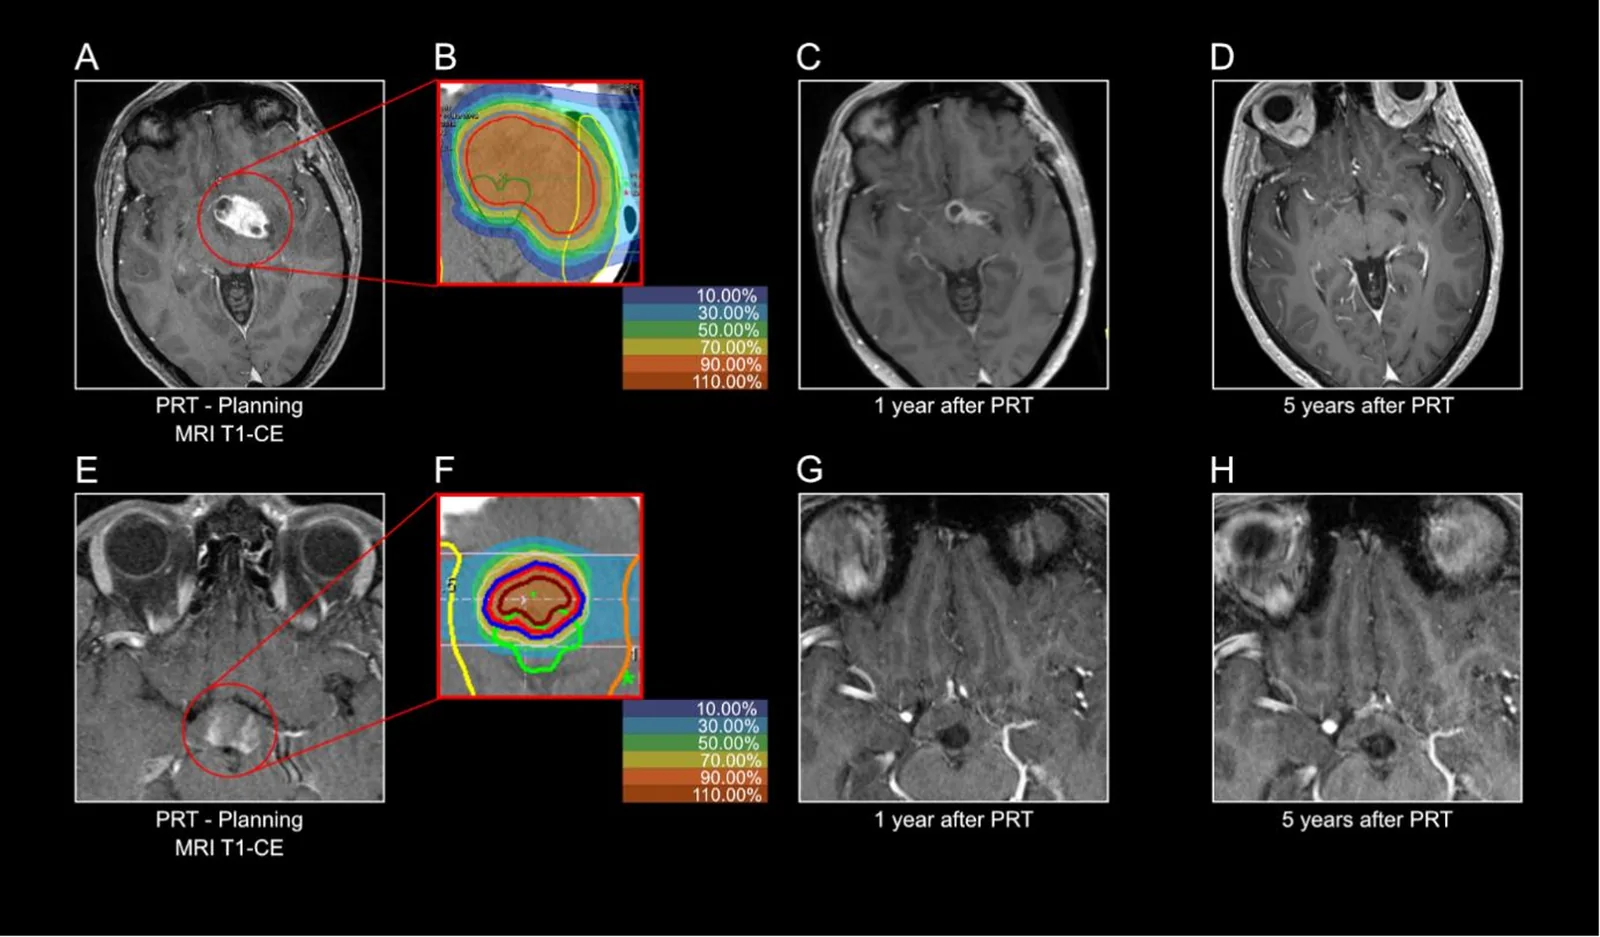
Representative longitudinal magnetic resonance imaging after proton radiotherapy. Two representative patient cases are shown (A-D: Astro-8, E-H: Astro-13). (A, E) Contrast-enhanced T1-weighted magnetic resonance images obtained for proton radiotherapy (PRT) planning, demonstrating the target lesions. (B, F) Corresponding treatment-planning images with target-volume contours and relative isodose distributions ranging from 10% to 110% of the prescribed dose, according to shown color-wash scale. (C, G) Follow-up contrast-enhanced T1-weighted images 1 year after PRT, showing marked radiographic regression of the treated lesions. (D, H) Long-term follow-up images approximately 5 years after PRT, demonstrating complete response. Abbreviations: PRT, proton radiotherapy; MRI, magnetic resonance imaging; CE, contrast-enhanced
